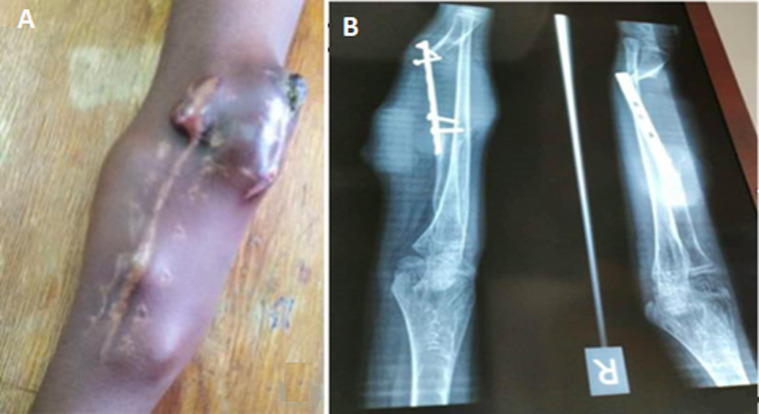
15-year-old boy with diaphyseal giant cell tumor: A) multiple masses on right forearm with the most prominent at the proximal aspect of the antecubital fossa; B) radiograph of the right forearm; although image of poor quality, a relatively intact epi-metaphysis of the distal radius can be noticed; the soft tissue shadowing on a previously resected diaphysis with lytic like features and distal screws pullout are identified
